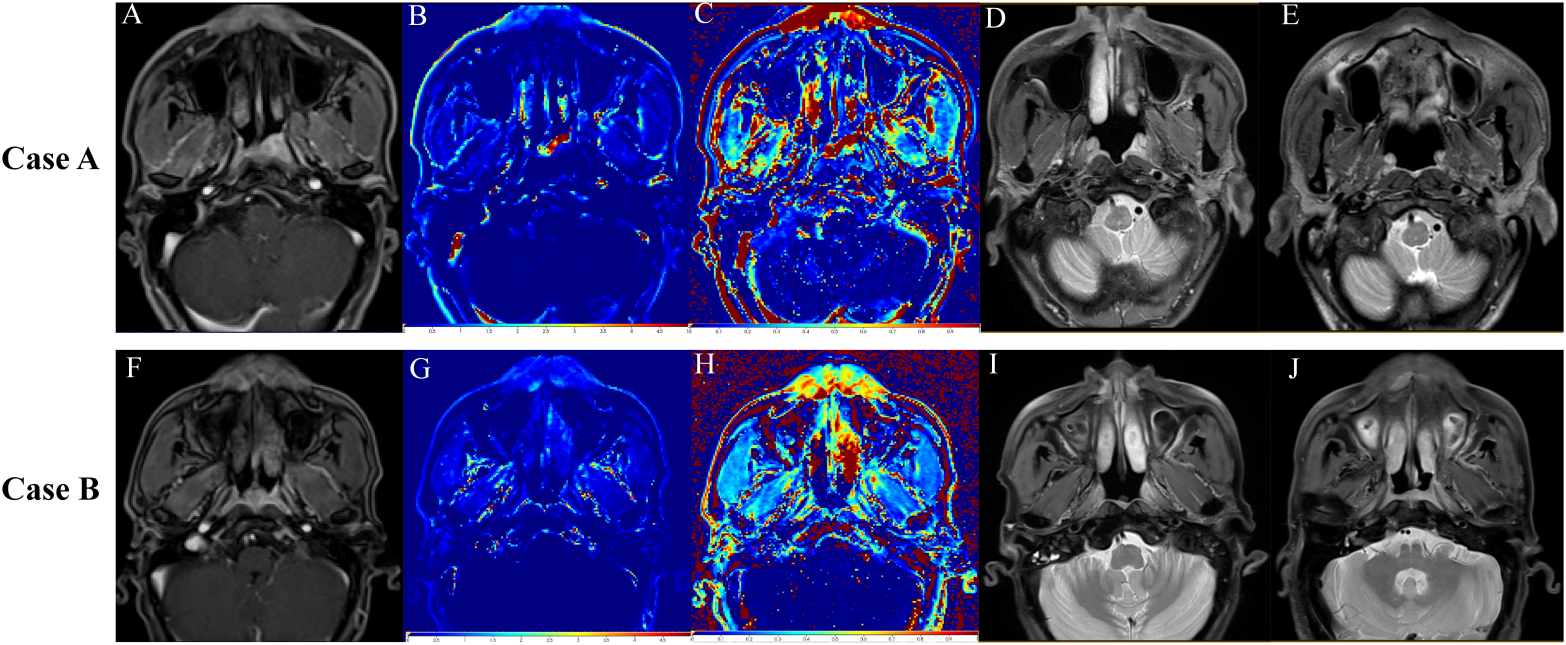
Representative images from three different time points during the follow-up period. The upper row shows images from Case A (T3N2M0) treated with nimotuzumab. (A–C) Baseline DCE-MR images. (A) Slice image. (B)
